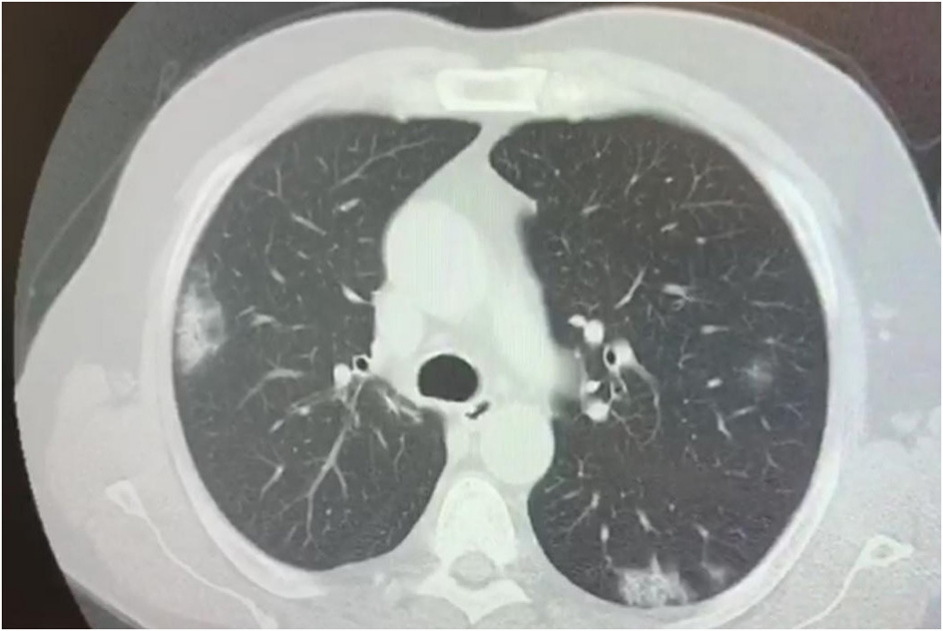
Chest CT scan showing bilateral consolidations in a 17 year old boy affected by SARS-CoV-2.
